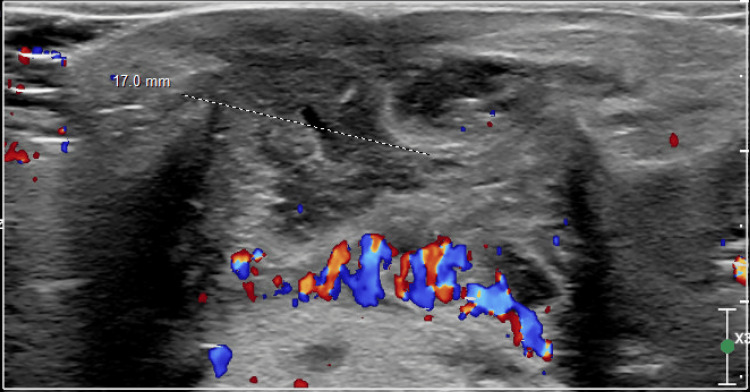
Ultrasound Demonstrating the Presence of a Thyroglossal Duct Cyst (TGDC)
